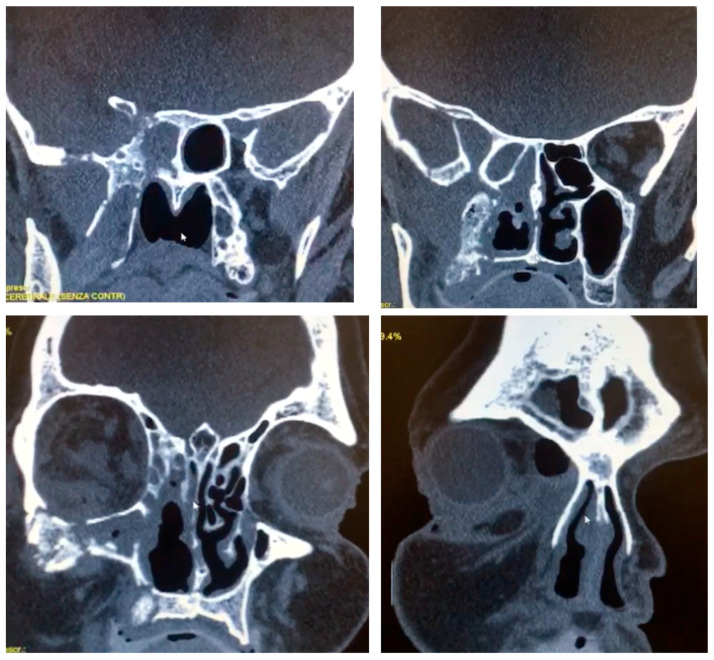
CT scan with contrast enhancement showing osteolytic changes involving the right maxillary walls, orbital floor, and medial orbital wall, reaching the sphenoid bone and skull base.
